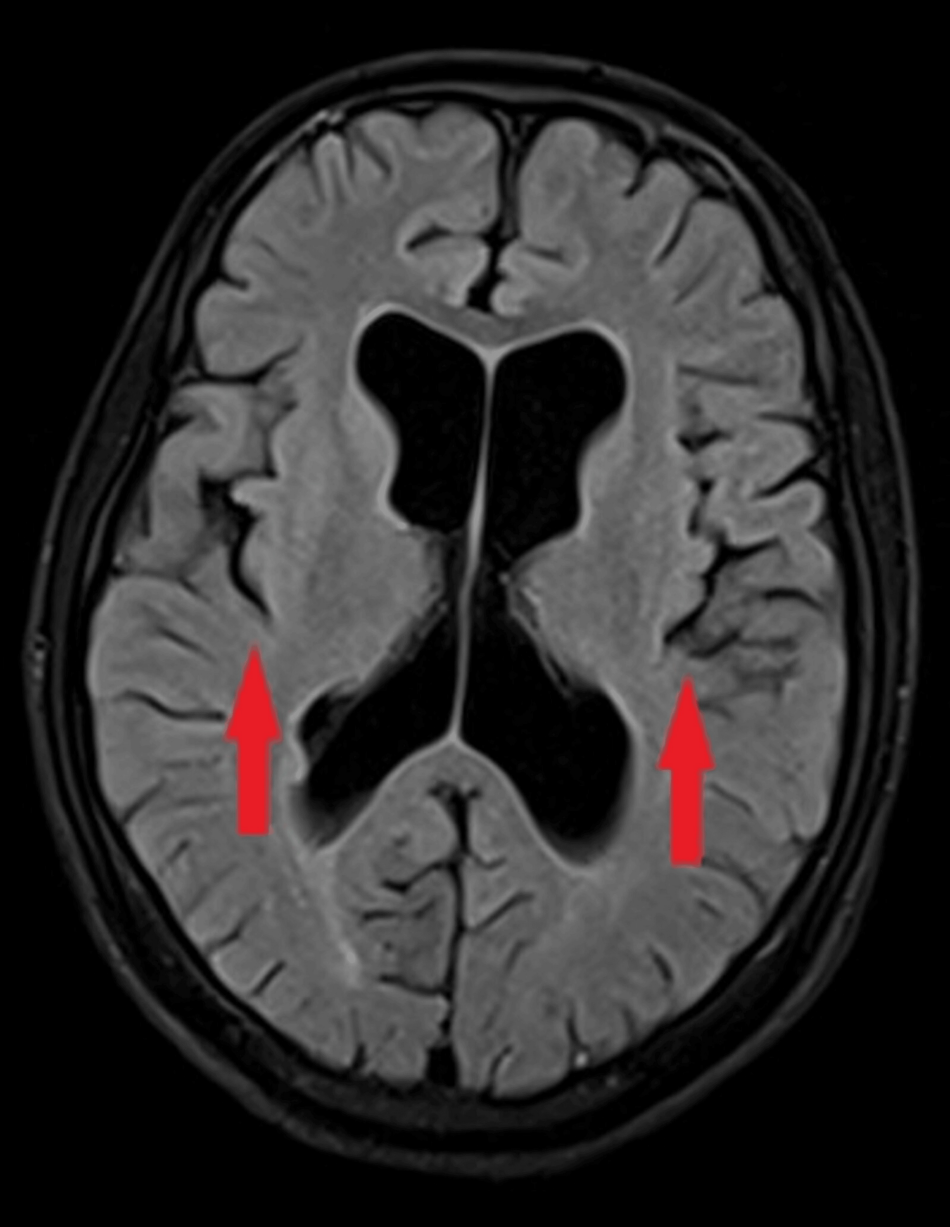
MRI of the brain using T2 FLAIR sequence showing diffuse cerebral atrophy (two red arrows). MRI: Magnetic resonance imaging; FLAIR: Fluid attenuated inversion recovery
